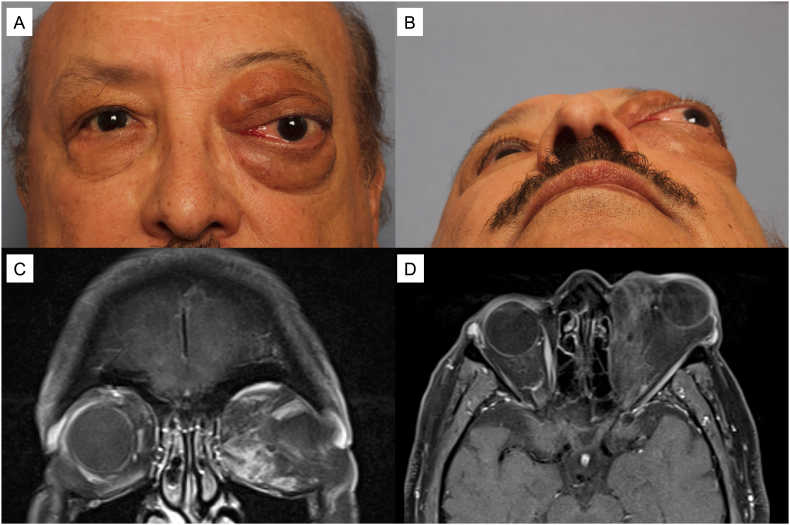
External images and MRI images of a 61-year-old patient with primary dedifferentiated liposarcoma of the left orbit. (A) There is significant left eye proptosis with surrounding edema of the upper and lower lid due to an irregularly shaped mass in the orbit. The photograph displays the patient examined in primary gaze. (B) Worm's eye view emphasizes the degree of proptosis and asymmetry. (C) T2-weighted magnetic resonance imaging of the orbit (coronal view) post contrast with fat suppression shows an irregular intraconal mass with variable areas of contrast enhancement. (D) Axial view.
